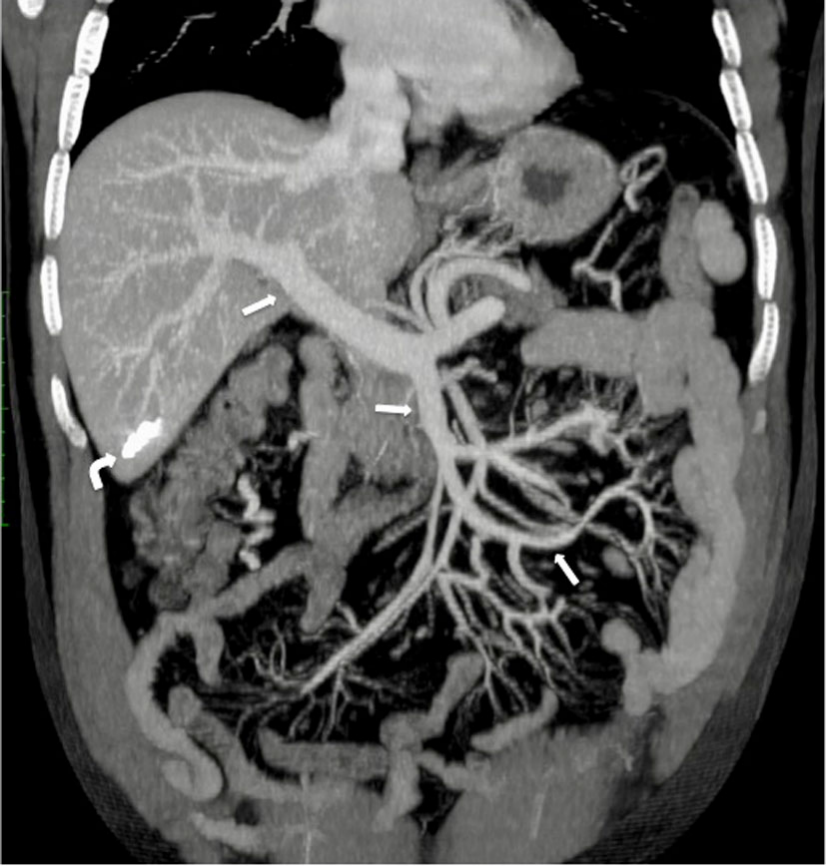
One-year follow-up CT shows complete recanalization of the SMV and PV (arrow); the hyperdense embolized hepatic tract is still visible within the sixth hepatic segment (curved arrow)
